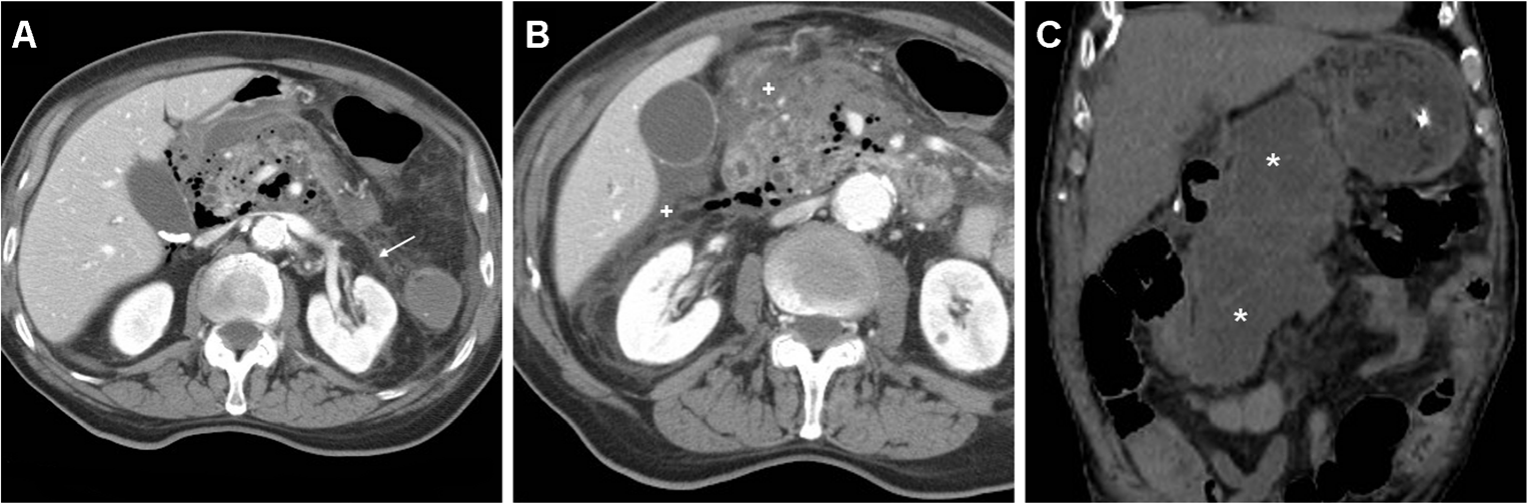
Necrotising AP plus retroperitoneal air in a 79-year-old male occurring 48 h after ERCP treatment of choledocholithiasis, including sphincterotomy. Contrast-enhanced CT (a, b) showed an enlarged, markedly inhomogeneous, poorly enhancing pancreas with fascial (*thin arrow* in a), peripancreatic and infrahepatic (+ in b) fluid, gas bubbles along the superior mesenteric vessels and in the periduodenal region. Pleural effusion was present bilaterally. Urgent laparotomic surgery confirmed severe necrotic-haemorrhagic pancreatitis. During his prolonged stay in the intensive care unit, follow-up unenhanced CT (c) showed the appearance of a vast walled-off postnecrotic collection (*)
